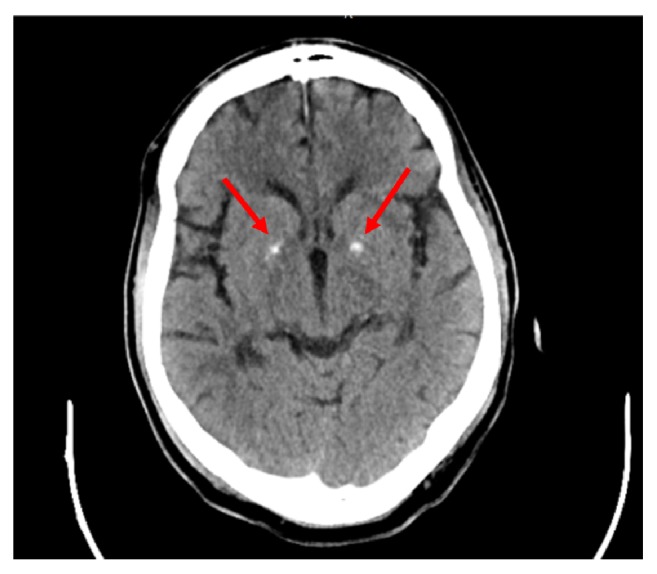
CT scan without contrast showing bilateral basal ganglia calcification.
